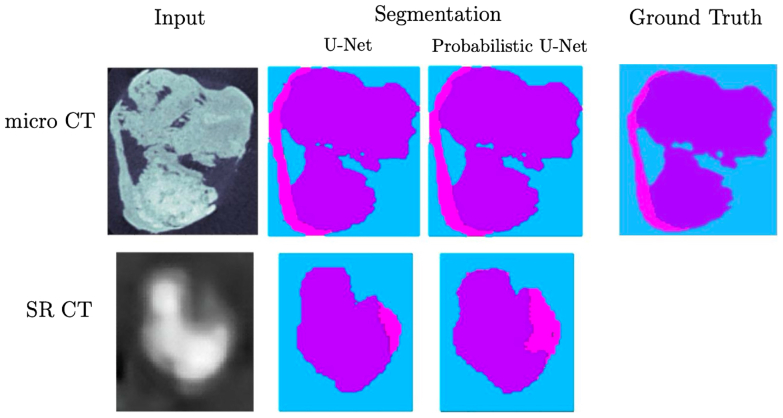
Example of a segmentation of the micro-computed tomography (CT) (first row) and its corresponding super-resolution-CT images (second row) with the U-Net and Probabilistic U-Net models (3 classes).
